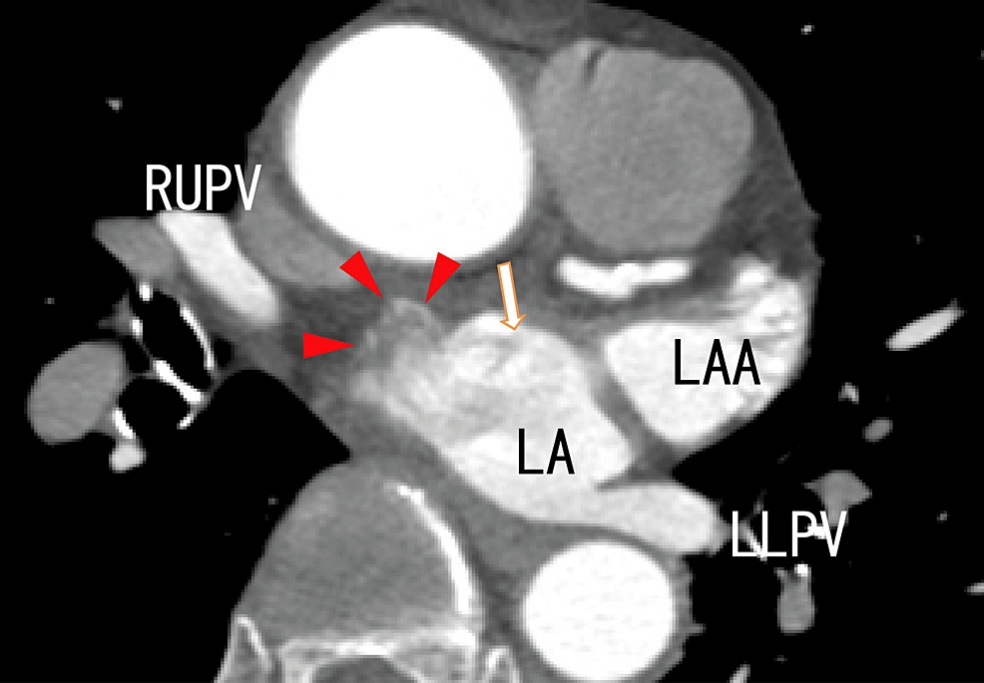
Axial images from an 80-MDCT scan revealing the RUPV, LLPV, and LA There was a rather dark area (arrow), and there were some images of thrombi in the LA as the dark area. There were some enhanced areas on the anterior wall of the LA wall (arrowheads), suggesting that the diverticulum was full of thrombi. LA, left atrium; LAA, left atrial appendage; LLPV, left lower pulmonary vein; RUPV, right upper pulmonary vein; MDCT: multidetector computed tomography
